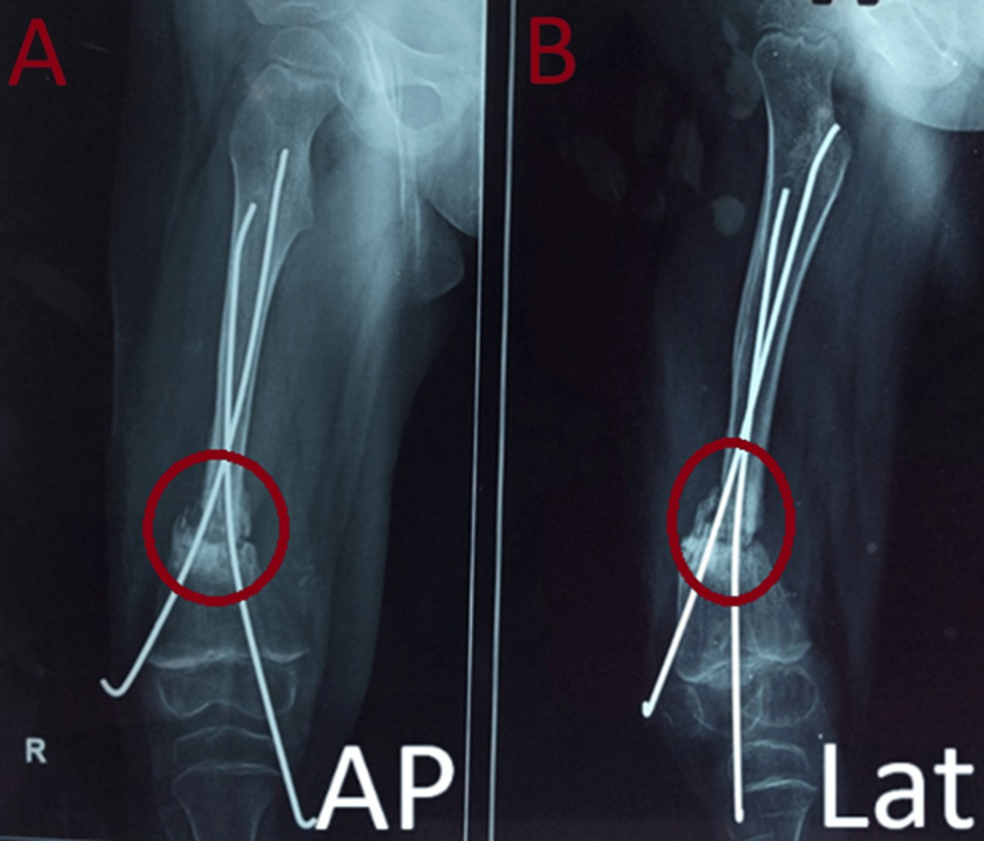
Third-month follow-up radiographs showing progressive signs of fracture union A: AP view, B: Lateral view
